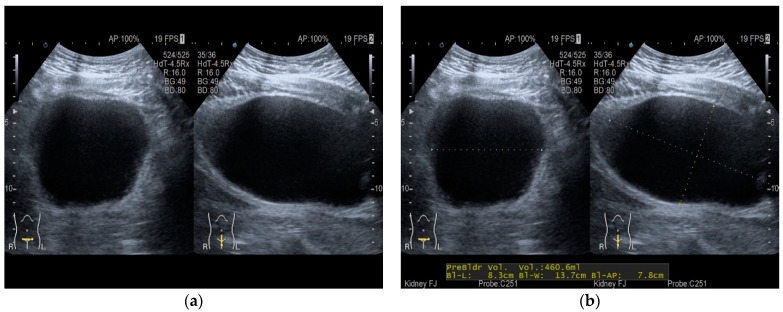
Traditional bladder volume estimation. (a) B-mode ultrasound of bladder, with transverse view (left) and sagittal view (right) shown. (b) Width is measured from a transverse view (left); height and length are measured from a sagittal view (right).
